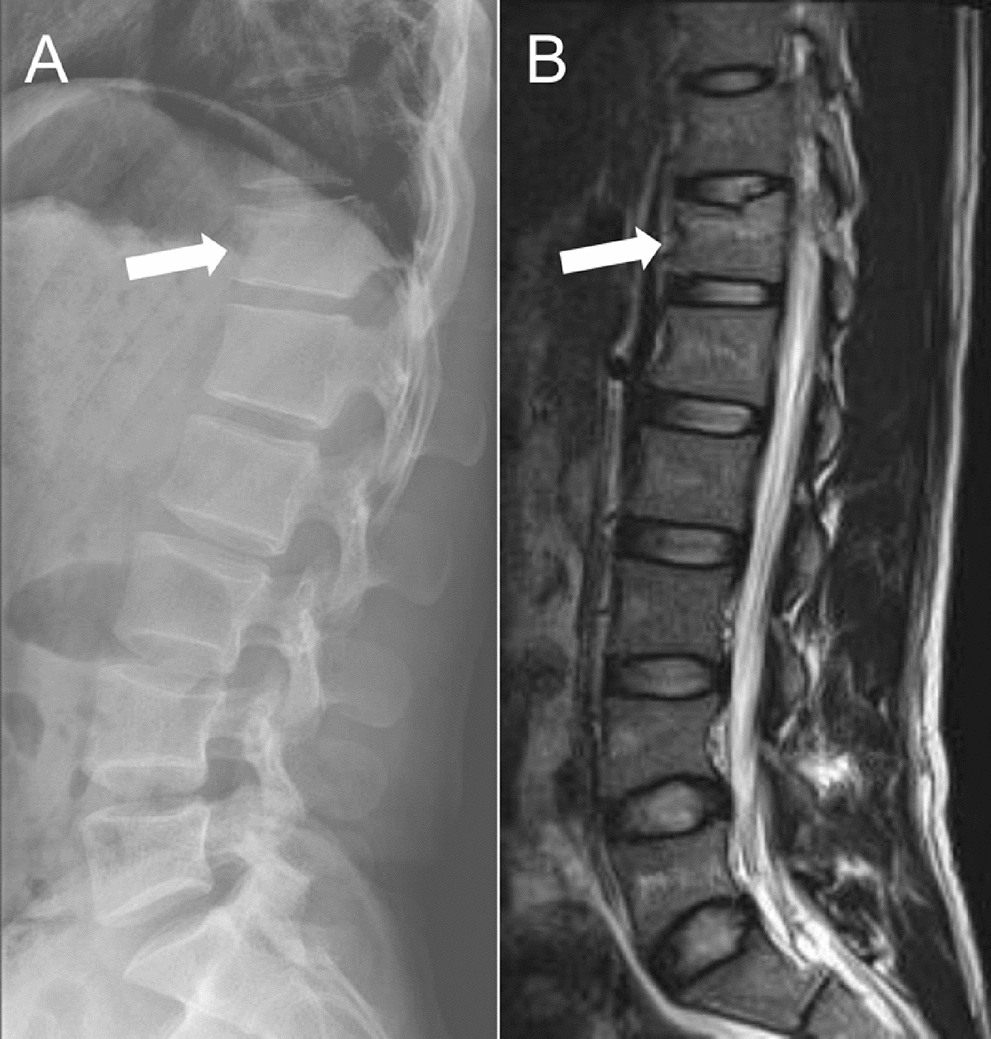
Preoperative imaging assessment of the T12 vertebral compression fracture. A An anteroposterior radiograph of the thoracolumbar spine reveals an anterior wedge compression fracture at T12 (arrow), without posterior bone displacement or compromise of the spinal canal. B Sagittal T2-weighted MRI of the same region confirms an acute T12 fracture, demonstrating bone marrow edema at the fracture site (arrow), with an intact posterior ligamentous complex and no evidence of epidural hematoma or neural compression.
